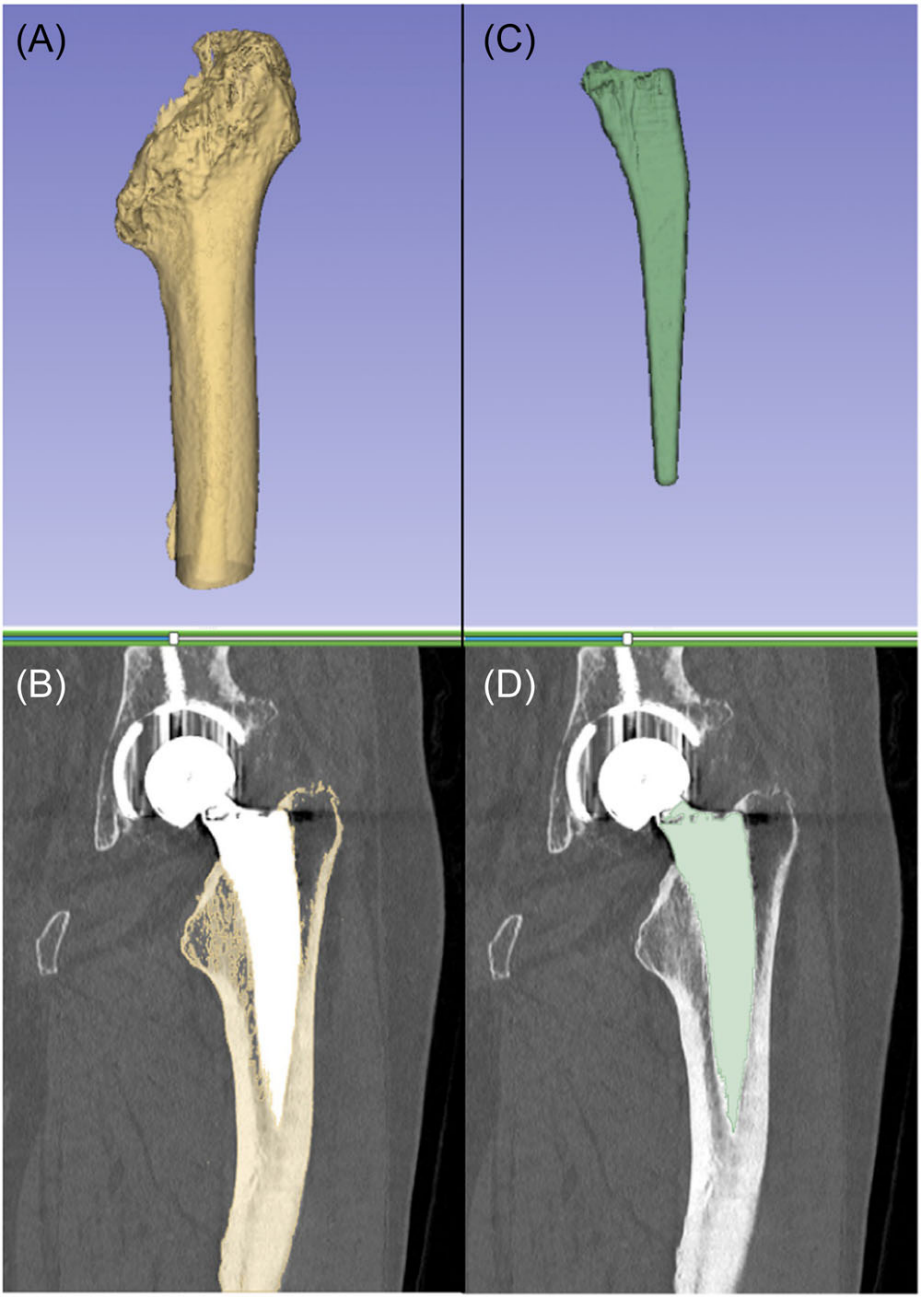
3D slicer results: (A) segmented three‐dimensional model of the femoral bone with (B) coronal in‐plane slice, and (C) segmented three‐dimensional model of femoral stem with (D) coronal in‐plane slice, creating the reference and migrating masks for CT‐RSA. CT‐RSA, computed tomography‐based RSA.
